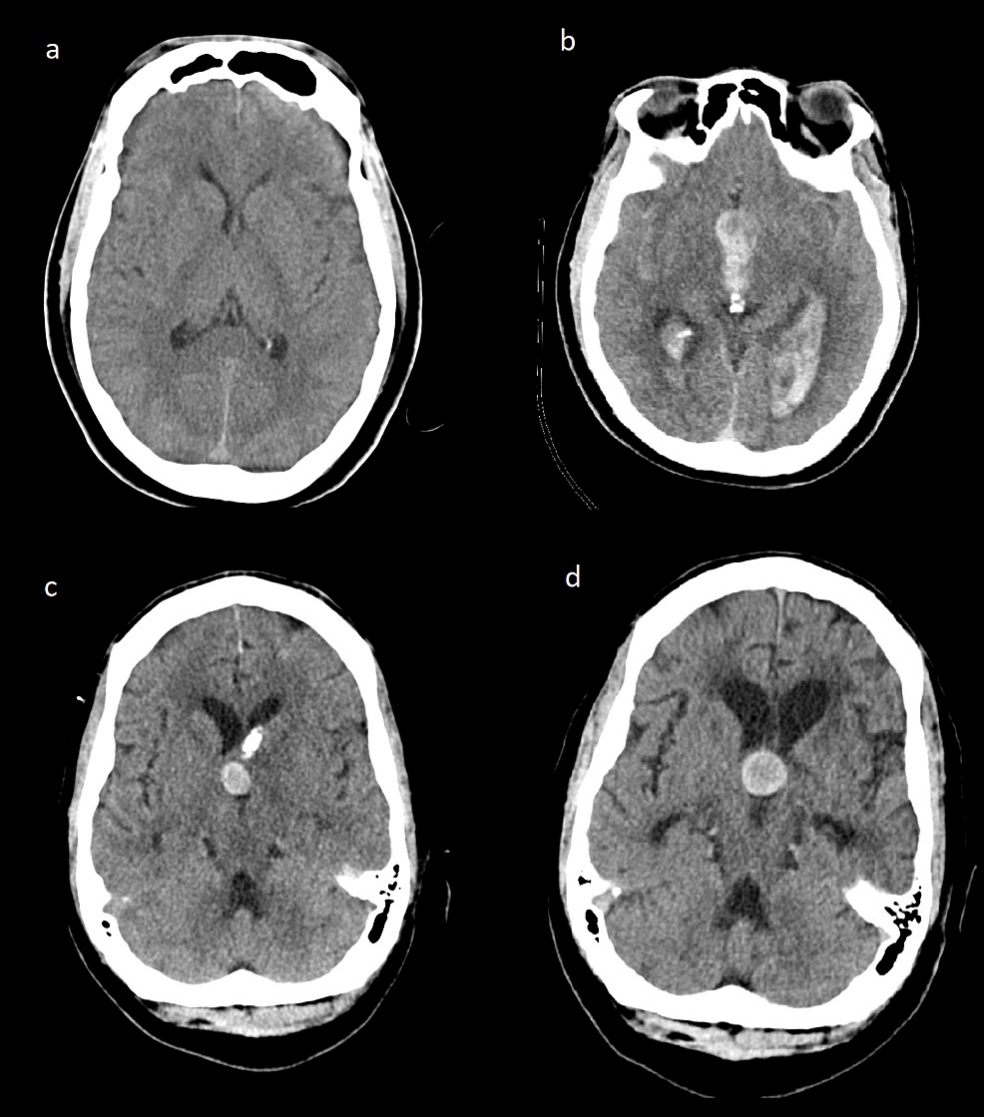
CT scan of the patient over 12 years of period CT head (a) May 27, 2008, without any intracranial abnormalities; (b) July 24, 2019, demonstrating intraventricular hemorrhage and hydrocephalus; (c) August 14, 2019, demonstrating resolution of IVH with evidence of third ventricular mass adjacent to the foramen of Monro and external ventricular drain; (d) November 25, 2020, demonstrating obstructive hydrocephalus secondary to a hyperdense third ventricular mass. IVH: intraventricular hemorrhage
